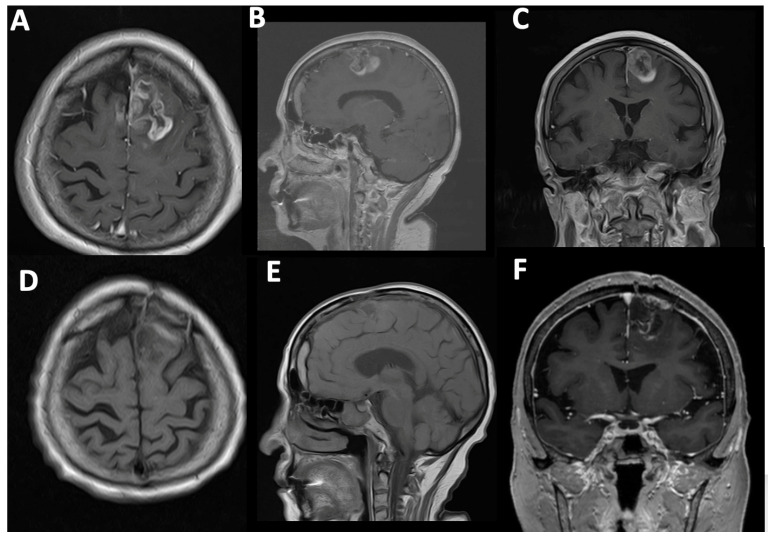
Fluorescent guided surgery. (A) A 54-year-old female first presented with a stroke suspicion, but after a detailed investigation, a left frontal HGG with relevant mass effect was revealed. Before the operation, the relatives also noticed signs of memory loss. Neurological examination revealed right-sided hemiparesis. (A–C) Preoperative T1-weighted contrast-enhancing images showing left frontal HGG. (D–F) Postoperative T1-weighted contrast-enhancing images showing no contrast enhancement. (B,E) Note the chronic subdural hematoma.
